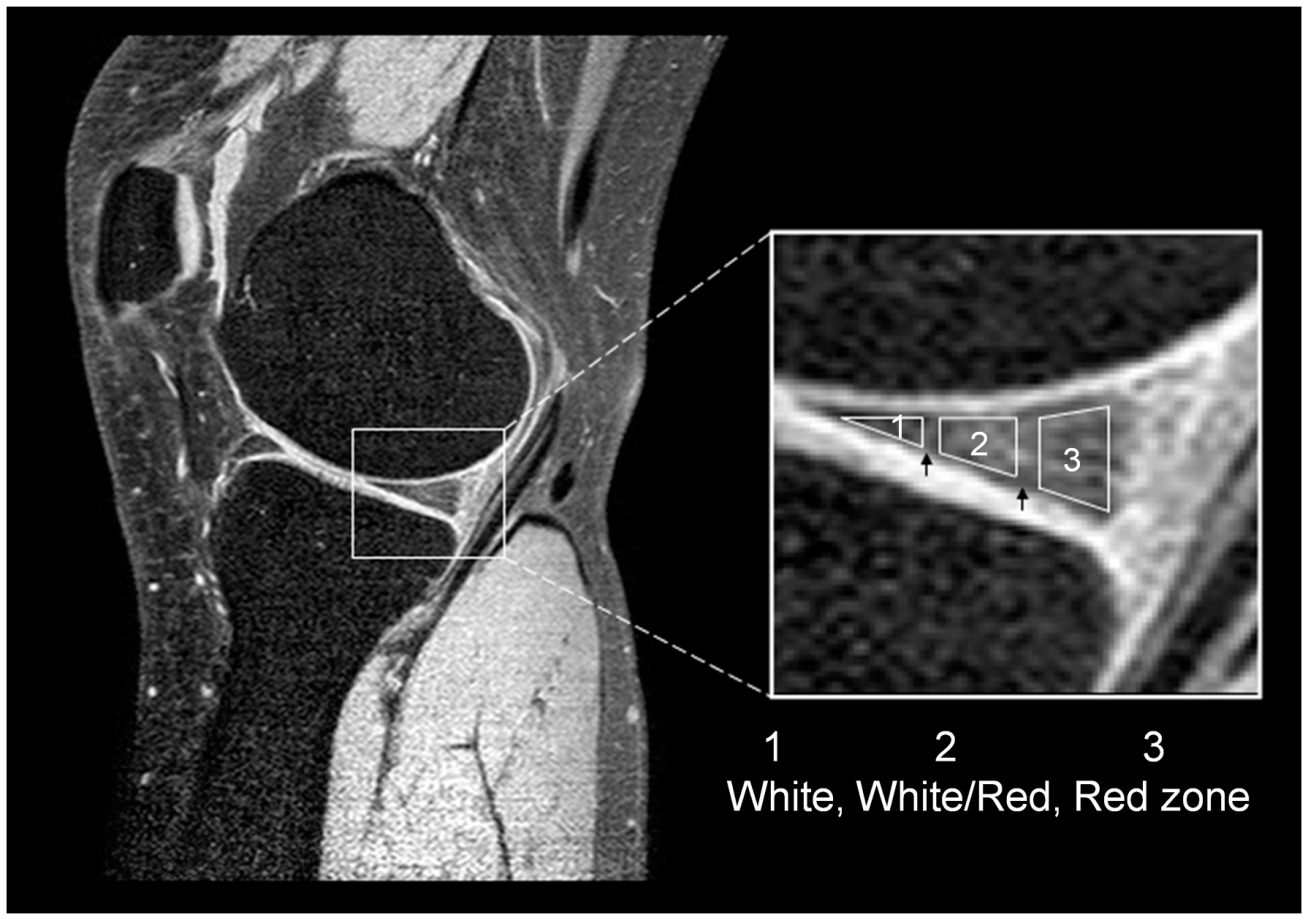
Representative ROIs in a nearly sagittal slice of a knee meniscus are shown. The selection of ROIs was performed on the first-echo MR image, which is enlarged on the right side. The black arrows indicate the separation between the three zones, and each zone was about one-third the width of the meniscus.
